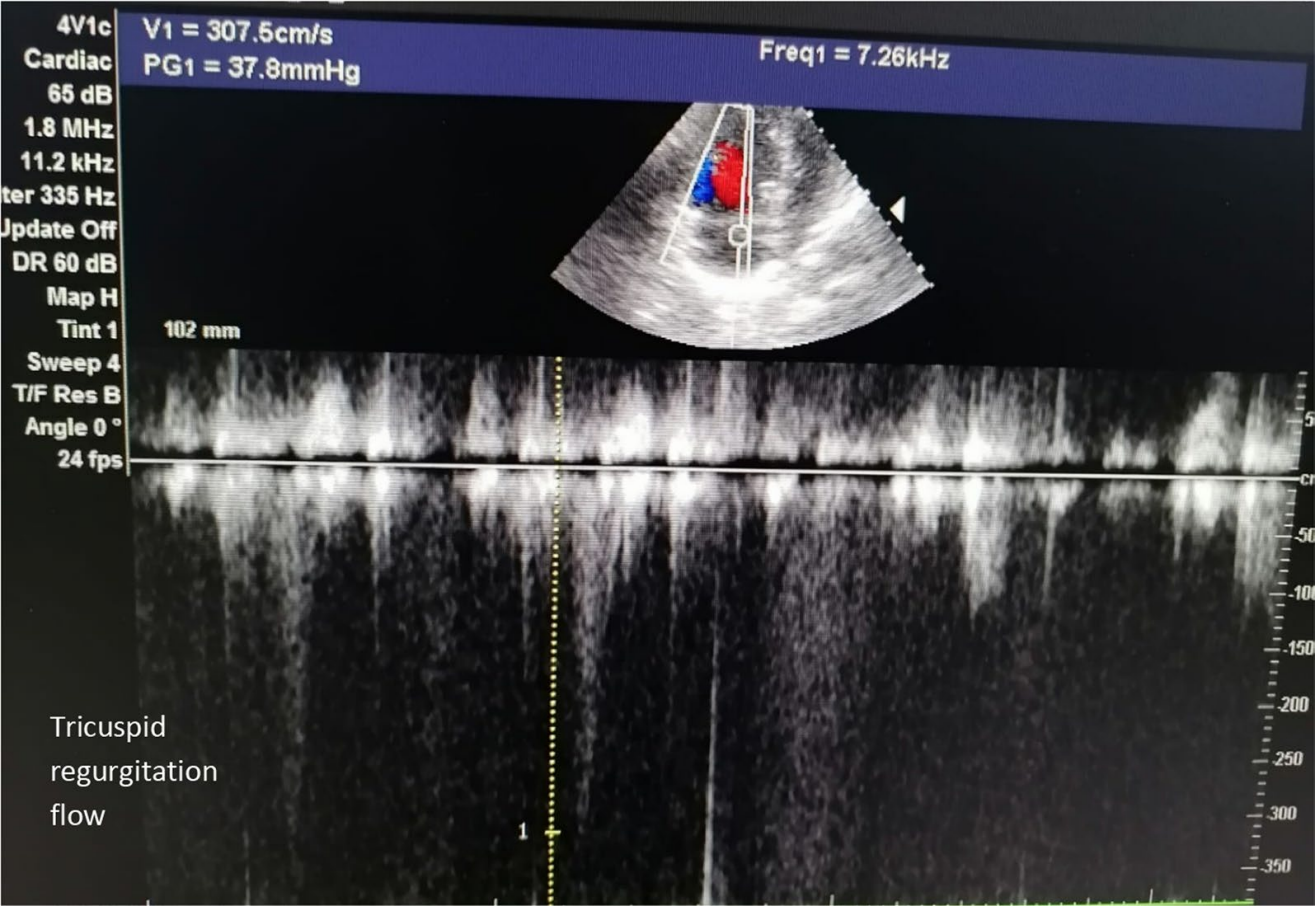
Patient echocardiogram at ER showing mild tricuspid regurgitation and estimated right ventricular systolic pressure of about 53 mmHg
